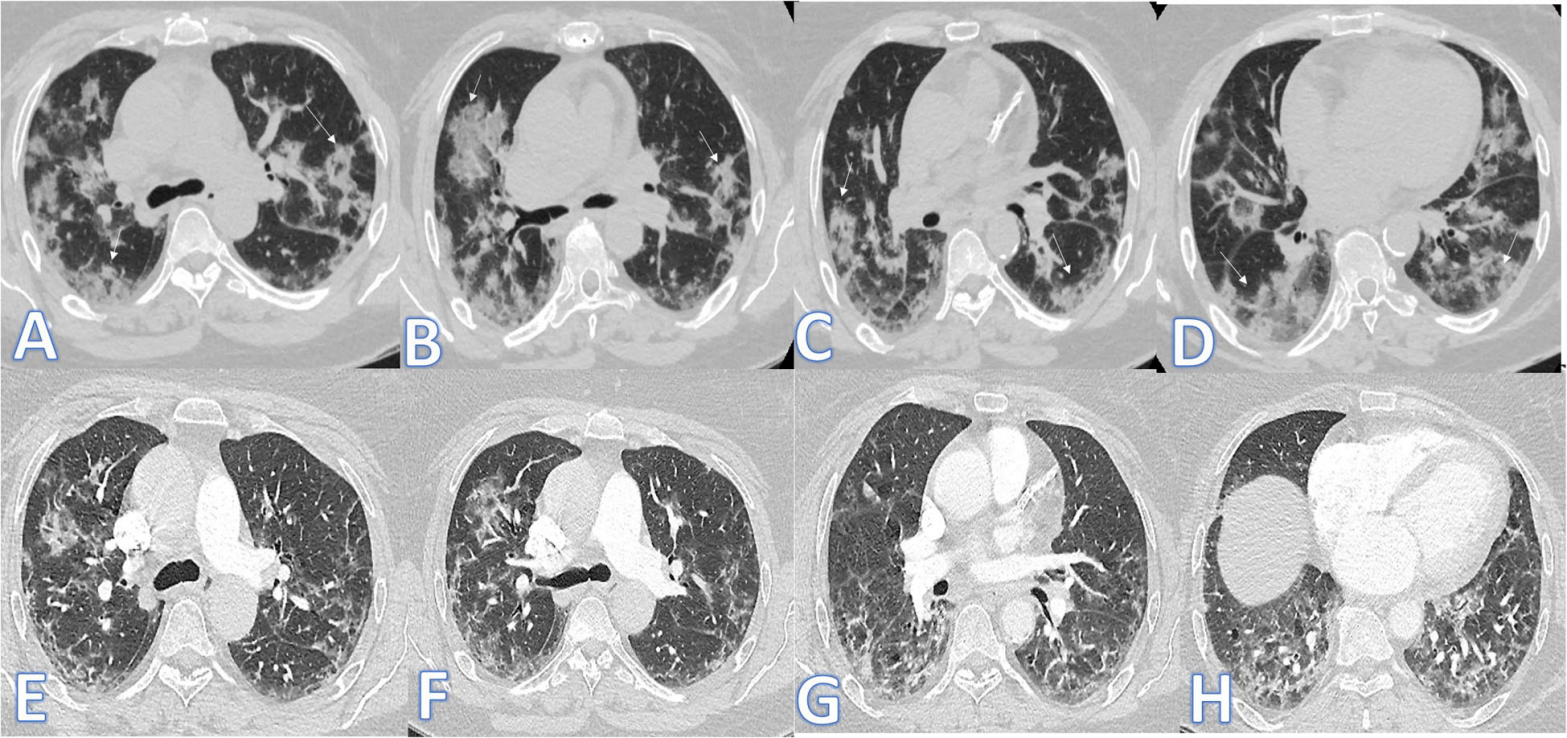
Fifty-five-year-old female: she has DM, HTN, and IHD; peak CT severity score 12; consolidation/crazy-paving score 10. She was admitted to the ICU; laboratory results show lymphopenia, high CRP, d-dimer, serum ferritin, and high-sensitivity troponin. Steroid was given; length of stay during hospitalization is 15 days. Peak CT during admission (a–d) showed bilateral consolidation patches more at the lower lobes with a crazy-paving appearance (arrows). Follow-up CT 3 weeks after discharge (e–h) shows lung fibrosis with a coarse reticular pattern mainly dependent (arrows).
